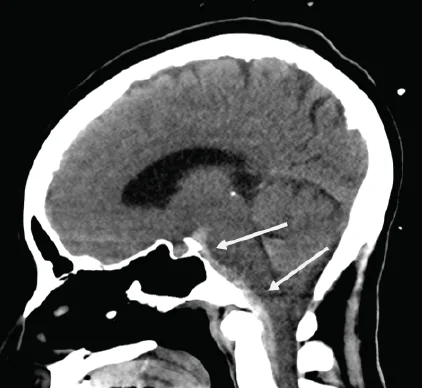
Computed tomography of the head (sagittal view) demonstrating hyperattenuation within the prepontine cistern, which extends inferiorly to the upper cervical spine relating to subarachnoid hemorrhage (arrows) in a patient diagnosed with perimesencephalic nonaneurysmal subarachnoid hemorrhage.
